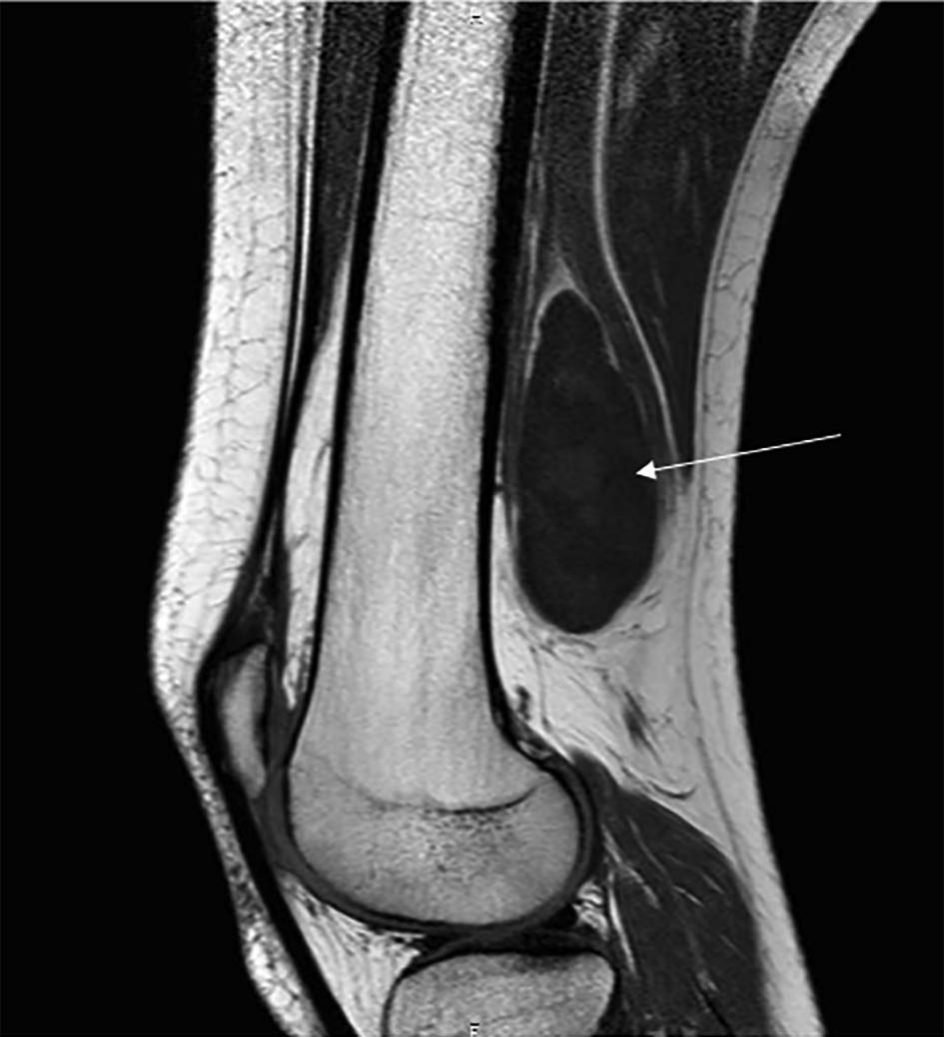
A sagittal T1-weighted MRI shows a well-defined mass, low signal intensity, inside the sciatic nerve, just above the popliteal fossa.
